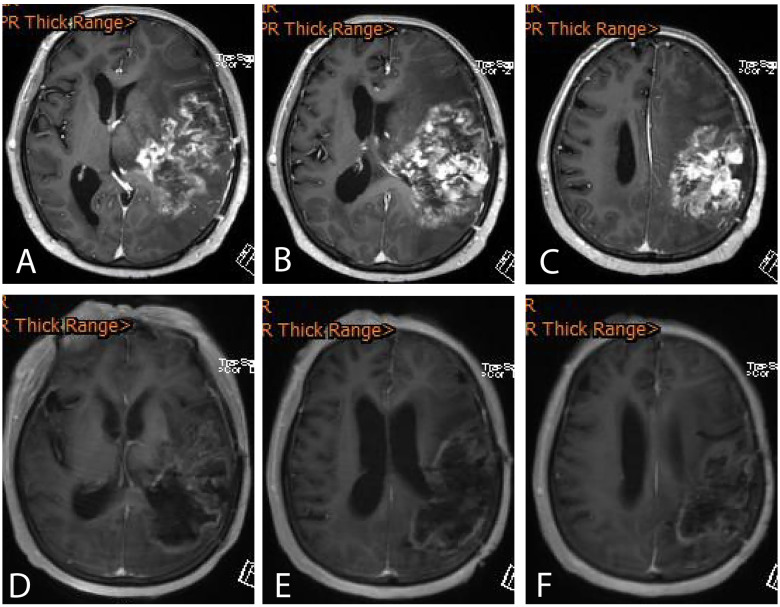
Postoperative follow-up of the case shown in Figure 1 by MRI. (A–C) MR images before ^125^ I brachytherapy. (D–F) At 6-month follow-up, contrast-enhanced T1W images demonstrate marked decreases in tumor enhancement, edema, and midline shift.
